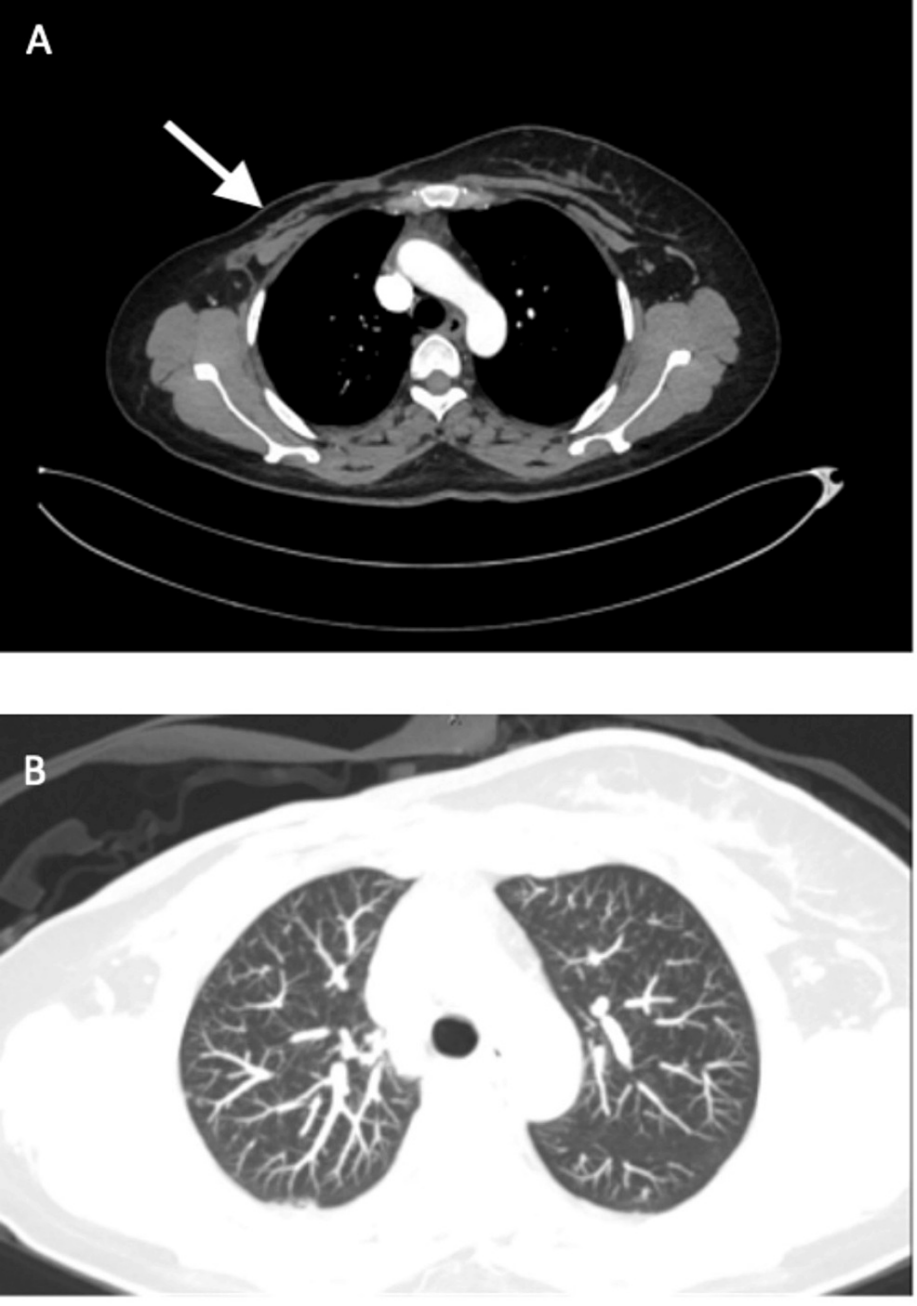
A horizontal section of computed tomography performed after three months of atezolizumab and nab-paclitaxel showed complete resolution of the right chest wall mass as indicated by the arrow (A) and complete resolution of bilateral pulmonary metastatic disease (B)
